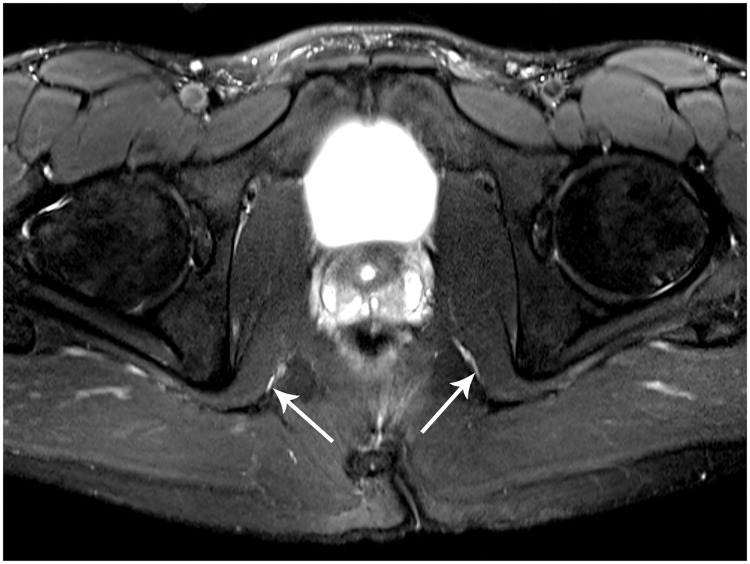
Post-operative pudendal nerve 3T MR neurography. Axial high spatial resolution T2-weighted MR image with fat suppression demonstrate near complete resolution of pudendal nerve engorgement (arrows) and decreased signal hyperintensity, consistent with interval improvement of symptoms.
